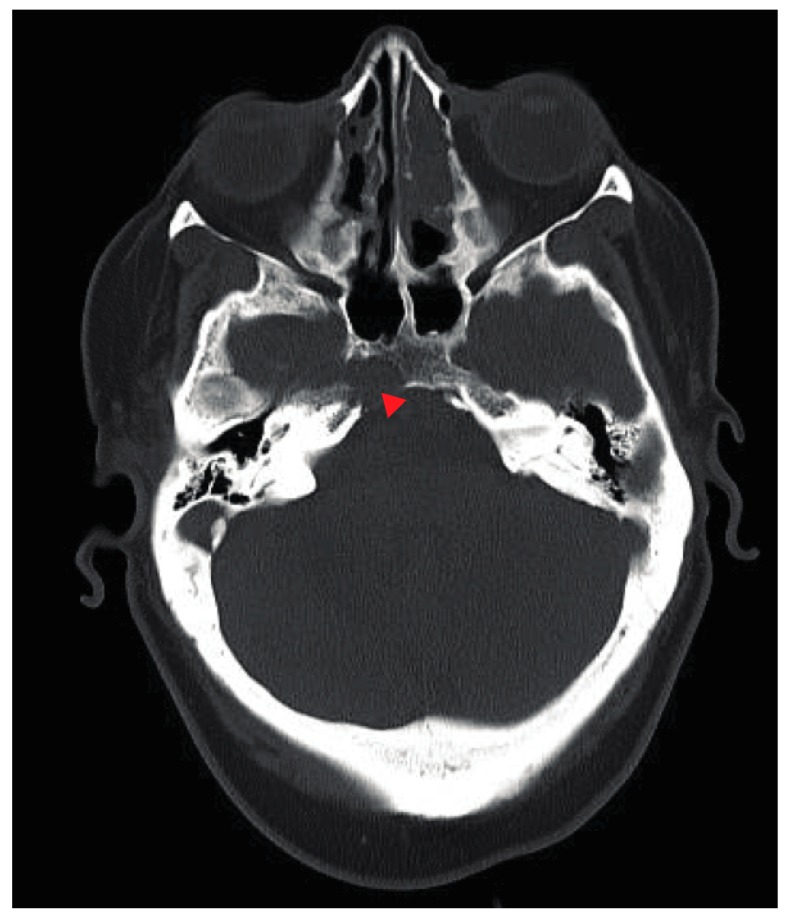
Axial CT head demonstrating lytic bone lesion (indicated by red triangle) within the right side of the clivus adjacent to Dorello’s canal likely accounting for the patient’s 6th nerve palsy.
